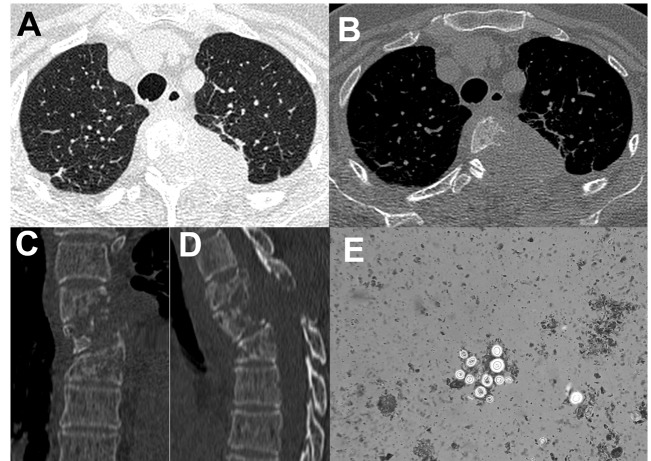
Chest CT with axial images of the lungs (A) and the bone
